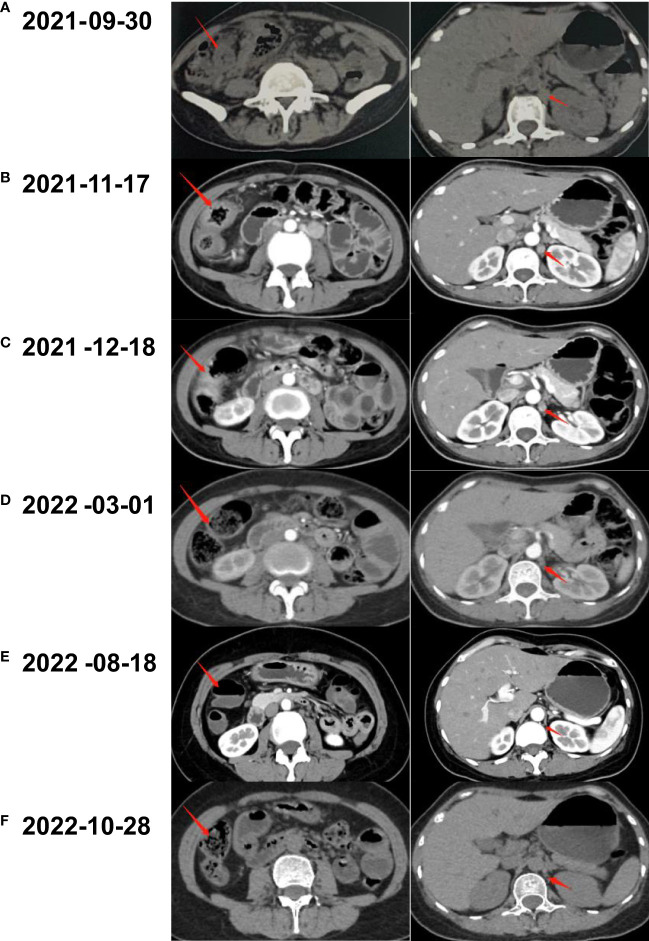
Abdominal CT scanning of the patient. (A) A neoplasm located in the right colon and thickened nodule in the retroperitoneal were shown by CT before treatment. (B) After three cycles of the combination of mFOLFOX6 and bevacizumab, CT demonstrated shrunken tumors in abdomen. (C) The lesion of colon and nodular did not continue to regress after another two cycles of chemotherapy plus targeted therapy. (D) CT scan revealed that the lesions came to gradual diminishment after eight cycles of envafolimab. (E) Thirty-six cycles of envafolimab later, the lesion in the right colon completely disappeared. (F) The patient kept clinical complete response with the use of envafolimab 400mg every 3 weeks.
